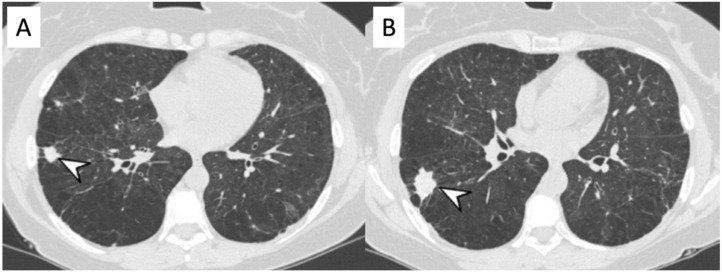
Peripheral nodular consolidations (arrowheads in (A,B)) located in the right lower lung of a female patient with eosinophilic granulomatosis with polyangiitis. Other pulmonary HRCT abnormalities, represented by emphysematous changes and not related to vasculitis, are also shown.
